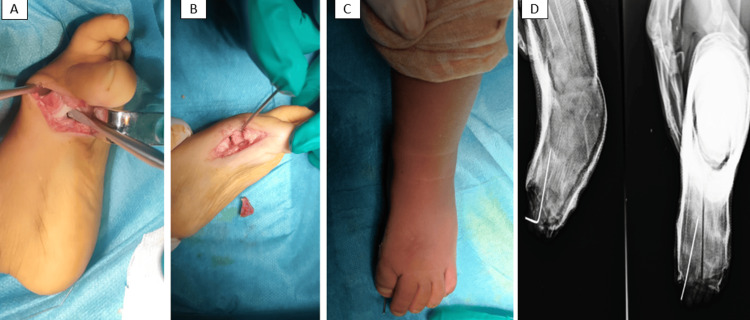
The surgical procedure A: Dorsomedial approach exposing the medial aspect of the foot, with resection of the abductor hallucis muscle (AbH). B: Capsulotomy of the first metatarsophalangeal joint followed by a valgus osteotomy of the first metatarsal. C: Stabilization of the metatarsophalangeal joint using a Kirschner wire. D: Immediate post-operative X-ray showing K-wires in situ.
